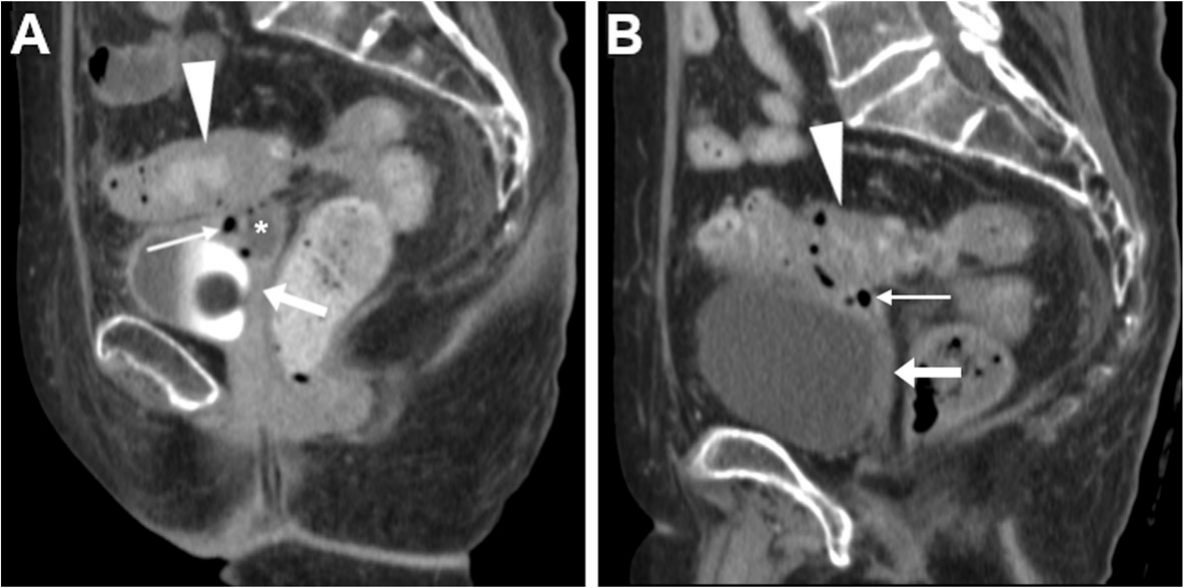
Surgically confirmed colo-VF in a 76-year-old with history of hysterectomy. During acute diverticulitis and *Clostridium difficile* colitis, CT (a) shows a small-sized abscess (*) at the vaginal dome (arrow), closely abutting the thickened walls of the sigmoid colon (arrowhead), which resolves after conservative treatment (b). Note air bubbles (thin arrows) in the vaginal stump
